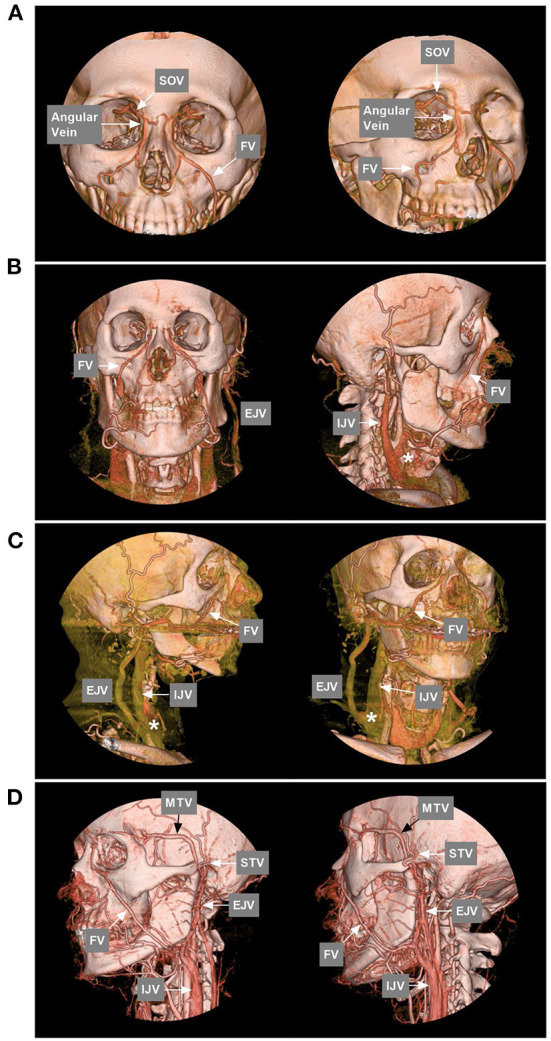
FV anatomy of CTA. (A) Anterior–posterior view (left) and oblique (right) view of CTA show that the SOV from the CS connected with the angular vein; then, the angular vein continued as the FV. (B) Anterior–posterior view (left) and oblique (right) view of CTA show that the typical FV descends obliquely in a straight line to continue the common FV and flow with the IJV. The asterisk (right) indicates the junction of the FV with the IJV. (C) Lateral view (left) and oblique (right) view of CTA show the FV together with the EJV into the IJV. The asterisks (left and right) indicate the junction of the FV into the IJV. (D) Lateral view (left) and oblique (right) view of CTA show that the MTV joins the STV to form the EJV and then connects with the FV into the IJV; in the face, many tidy veins join into the FV. CS, cavernous sinus; CTA, computed tomography angiography; EJV, external jugular vein; FV, facial vein; IJV, internal jugular vein; MTV, middle temporal vein; SOV, superior ophthalmic vein; STV, superficial temporal vein.
